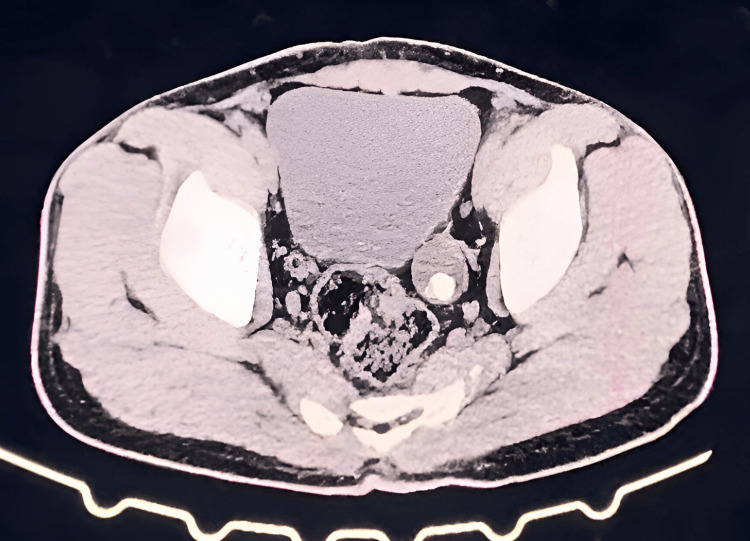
CT urogram showing dilated ureter with calculus
